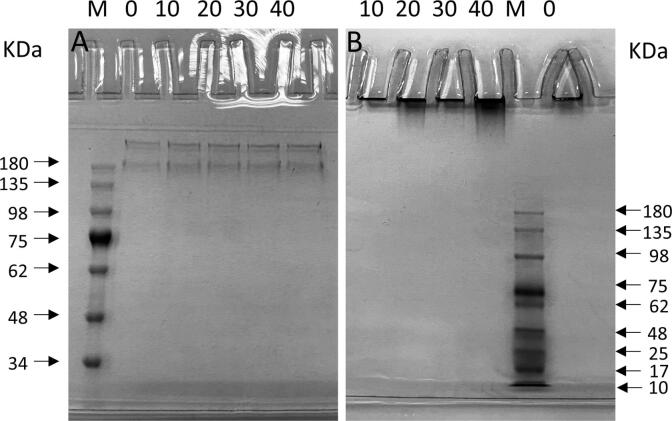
Effects of ultrasound treatment on the molecular weight of OVM. SDS-PAGE (A) and Native-PAGE (B).
